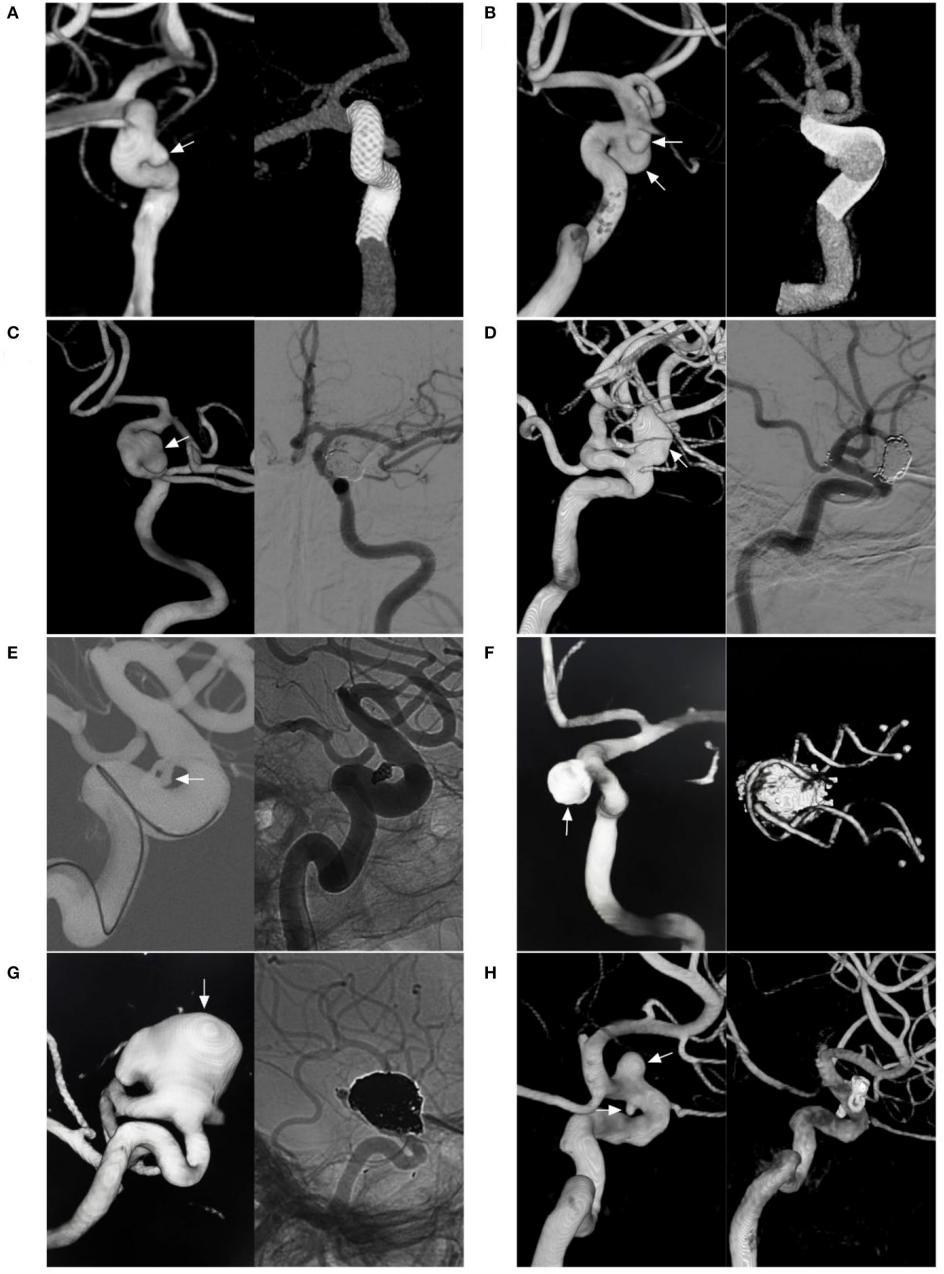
Various EVT techniques for aneurysms of the paraophthalmic segment. (A) Left, three-dimensional DSA shows a carotid artery aneurysm (arrow); right: Vaso CT shows the FDS covering the aneurysm. (B) Left, three-dimensional DSA shows two aneurysms (arrows) of the paraophthalmic segment; right: Vaso CT shows the FDS covering two aneurysms. (C) Left, three-dimensional DSA shows a clinoidal aneurysm (arrow); right: follow-up DSA shows that the aneurysms were coiled completely. (D) Left, three-dimensional DSA shows an OphA aneurysm (arrow); right: follow-up DSA shows that the aneurysm was coiled under stenting assistance. (E) Left, road map DSA shows a microcatheter into a paraclinoid-type SHA aneurysm (arrow); right: DSA shows the aneurysm was coiled. (F) Left, postoperative three-dimensional DSA shows a coiled suprasellar-type SHA aneurysm (arrow); right: Vaso CT shows the coils and stent. (G) Left, three-dimensional DSA shows a large OphA aneurysm (arrow); right: unsubtracted follow-up DSA shows that the aneurysm was coiled under stenting assistance. (H) Left, three-dimensional DSA shows OphA and SHA aneurysms (arrows); right: follow-up three-dimensional DSA shows that two aneurysms were coiled under stenting assistance. CT, computed tomography; DSA, digital subtraction angiography; EVT, endovascular treatment; FDS, flow-diverting stent; OphA, ophthalmic artery; SHA, superior hypophyseal artery; Vaso, vascular space occupancy.
